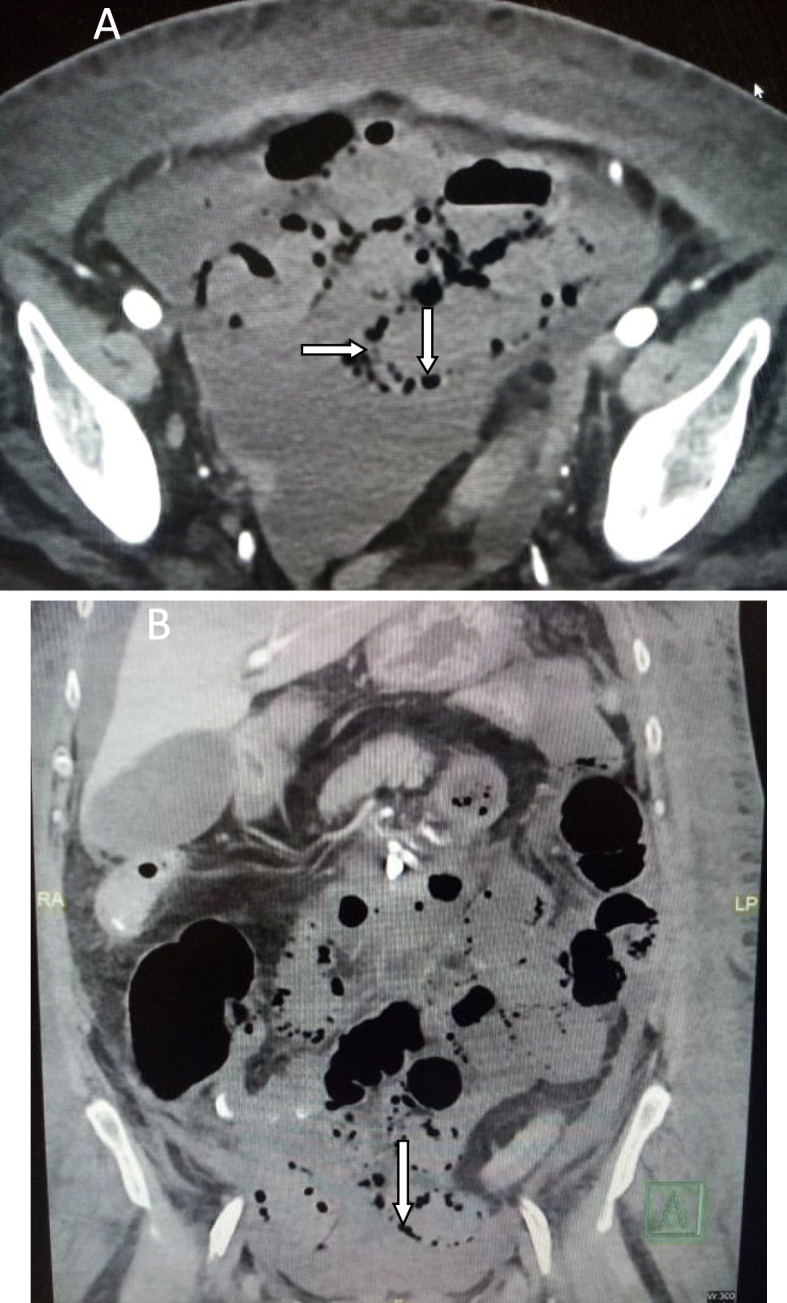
Seventy-year-old COVID-19 female patient isolated in the ICU presented with marked abdominal distention. a axial and b coronal CT of the abdomen show positive pneumatosis intestinalis (open arrows) and related collection
